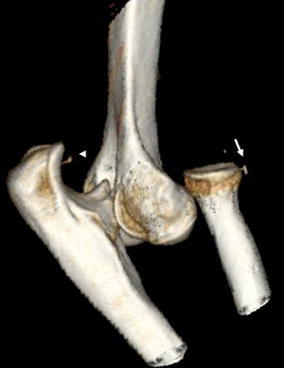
Lateral 3D reconstruction of CT scans of the *right elbow* confirm the divergent dislocation. Note the small radial head fracture. The coronoid process is abutting the posterior cortex of the medial humeral condyle. A tiny bone fragment off the coronoid process is seen between the olecranon fossa and humerus (*arrowhead*)
